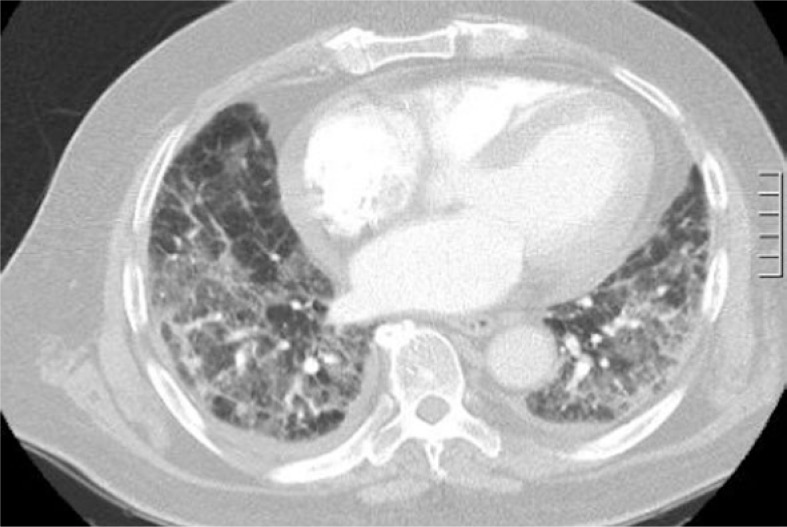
Representative image from CT pulmonary angiogram February 2009; diffuse infiltrates with interlobular septal thickening.
